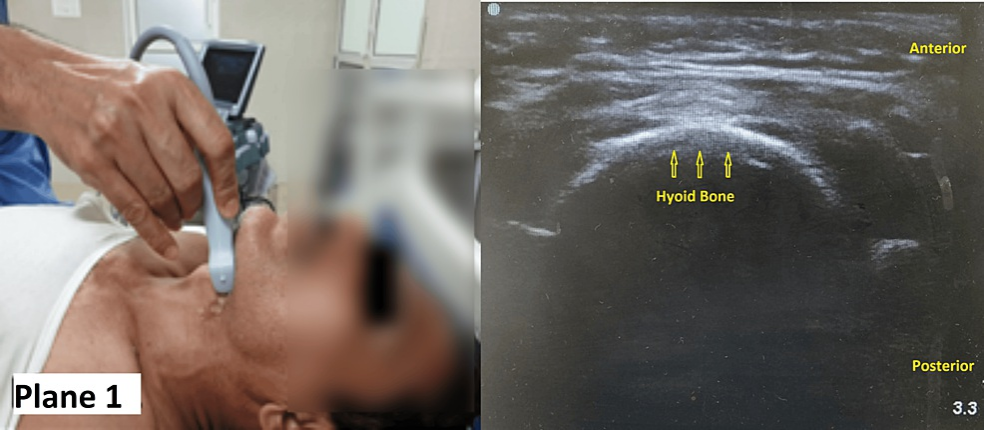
Measurement of the STT-Hyoid Note the position of the ultrasound probe on the neck (Plane 1) and the corresponding image STT-Hyoid: soft tissue thickness at the level of the hyoid
